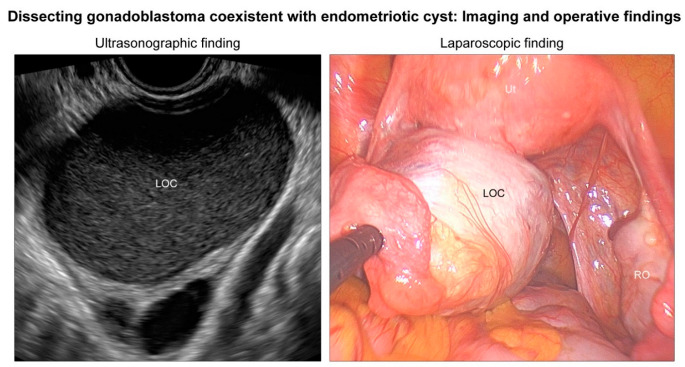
Imaging and operative findings. A 23-year-old woman presenting with intermittent low abdominal pain was referred to our institution with the possibility of an ovarian tumor. Preoperative ultrasonography revealed a 6.5 cm hypoechoic cystic mass in the left adnexa (LOC), suggestive of an ovarian endometriotic cyst. She underwent a laparoscopic left ovarian cystectomy. During laparoscopy, an endometrioma-like cystic mass was detected. The LOC contained chocolate-like fluid. The right ovary (RO) and uterus (Ut) were unremarkable except for a focal filmy adhesion between the anterior uterine serosa and peritoneum. No cul-de-sac obliteration was identified.
